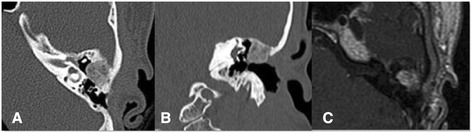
Computed tomographic scans and control magnetic resonance imaging study. Axial (a) and coronal (b) computed tomographic scans showing decreased attenuation and lytic images with bone rarefaction inside the epitympanum. c Axial T1-weighted magnetic resonance imaging scan with homogeneous contrast enhancement in the epitympanum
